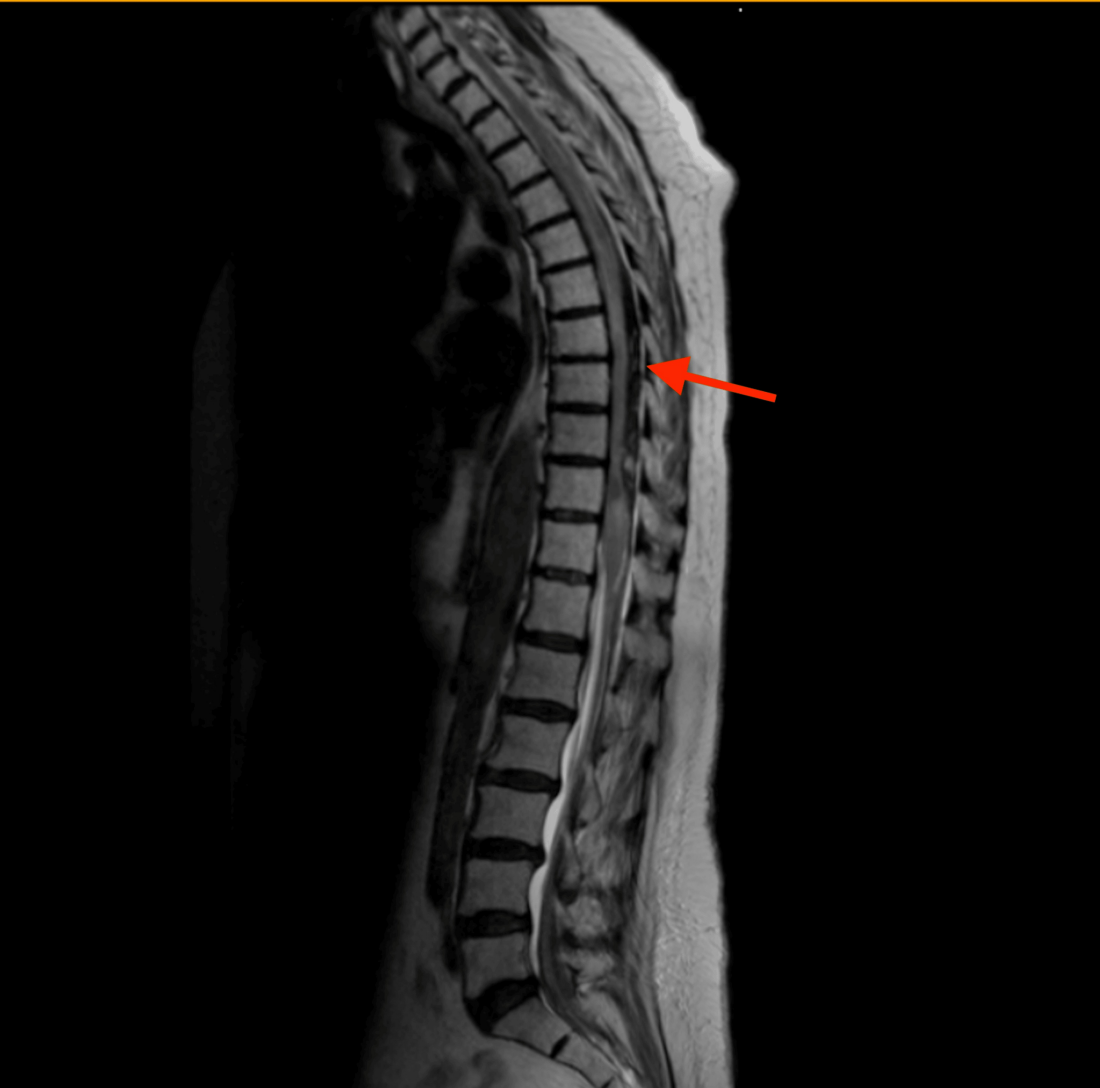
MRI of the thoracic spine showing 10 cm subdural hematoma MRI: magnetic resonance imaging
